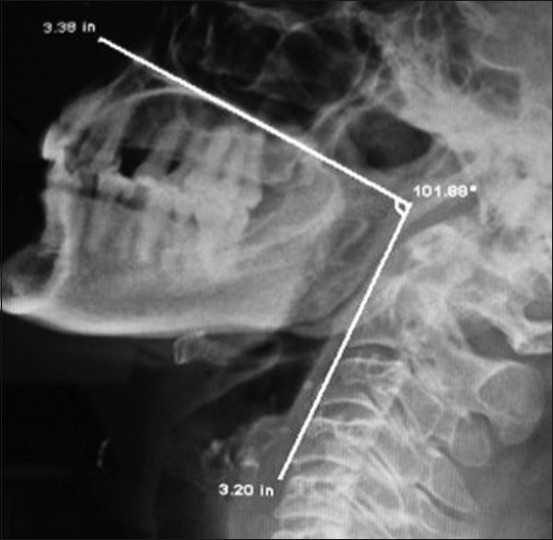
Radiograph of Neck-Lateral view showing the normal maxillo-pharyngeal angle > than 100 degree (Source: Department of Radio-diagnosis, Imaging and Interventional Radiology N.S.C.B.Subharti Medical College, Meerut)
